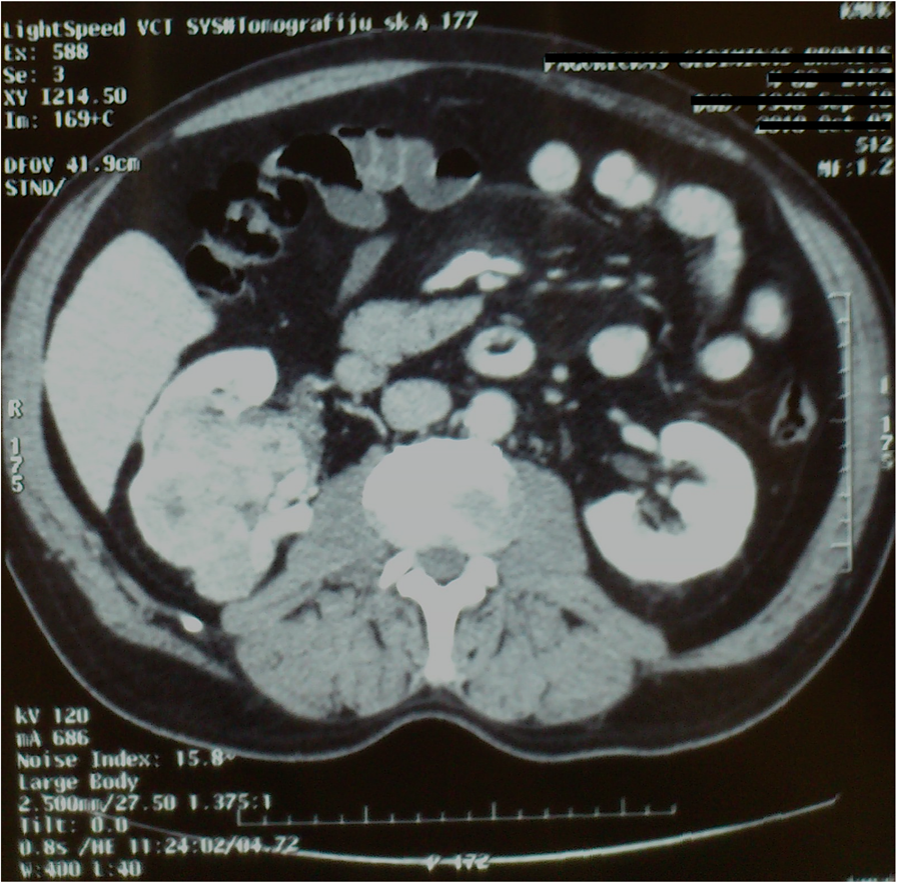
Preoperative abdominal computed tomography scan. The presence of renal tumor in the right kidney
